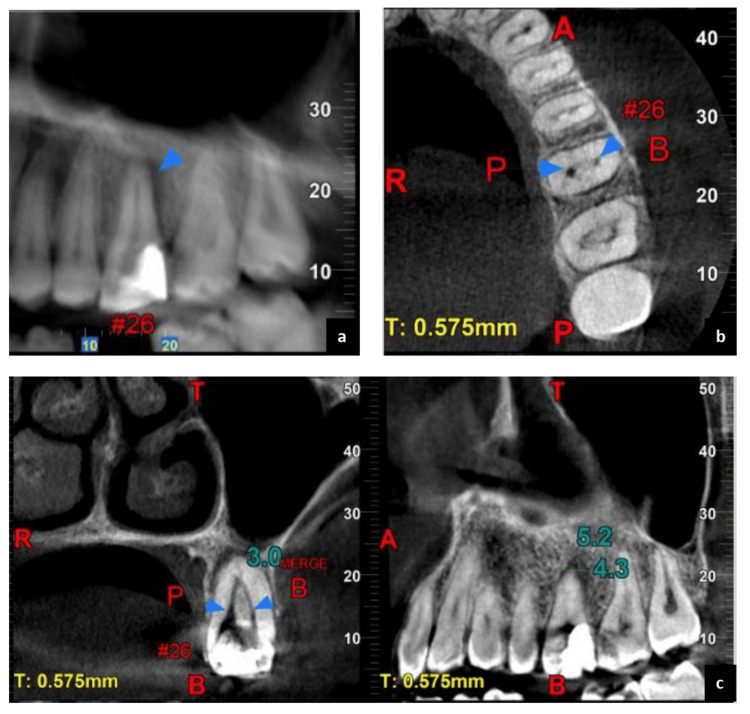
Cone beam computed tomography - (a) sagittal section, (b) axial section, and (c) coronal section.
